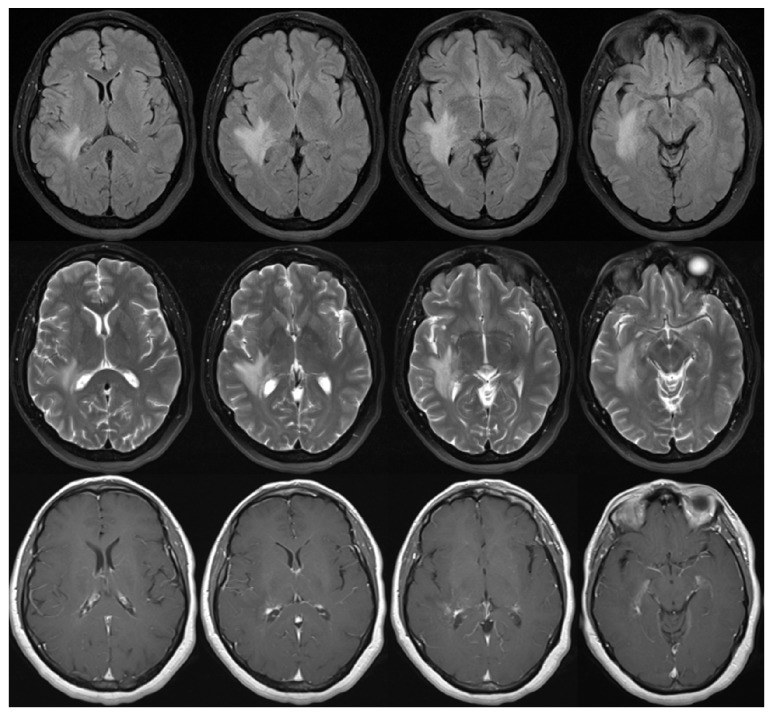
MRI of the brain showing peritrigonal (temporal lobe) deep white matter mass measuring 2.6 × 3.5 cm with surrounding vasogenic edema. There was no restricted diffusion with slightly increased flow at the affected area and normal cerebral blood volume on MR perfusion. Axial and sagittal T1 postgadolinium studies showed minimal enhancement.
